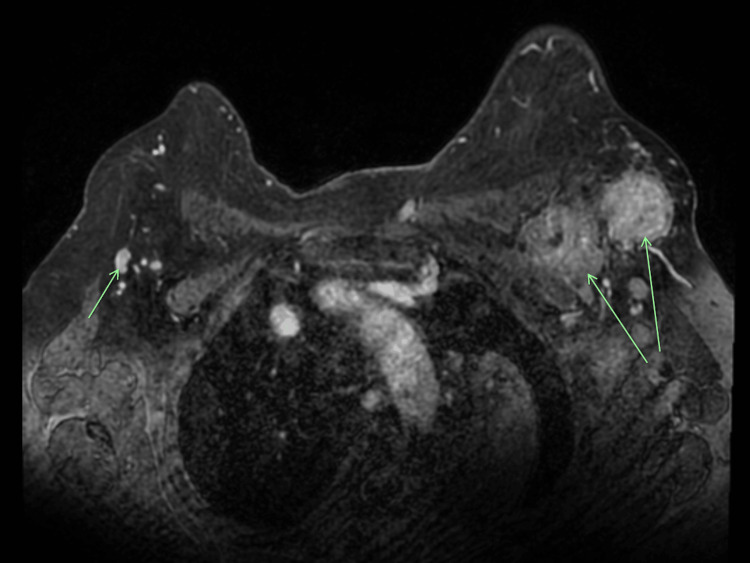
Axial view of MRI Axial view of MRI, demonstrating bilateral axillary lymphadenopathy (indicated with arrows), without evidence of pathological lesions in either breast. MRI, magnetic resonance imaging
